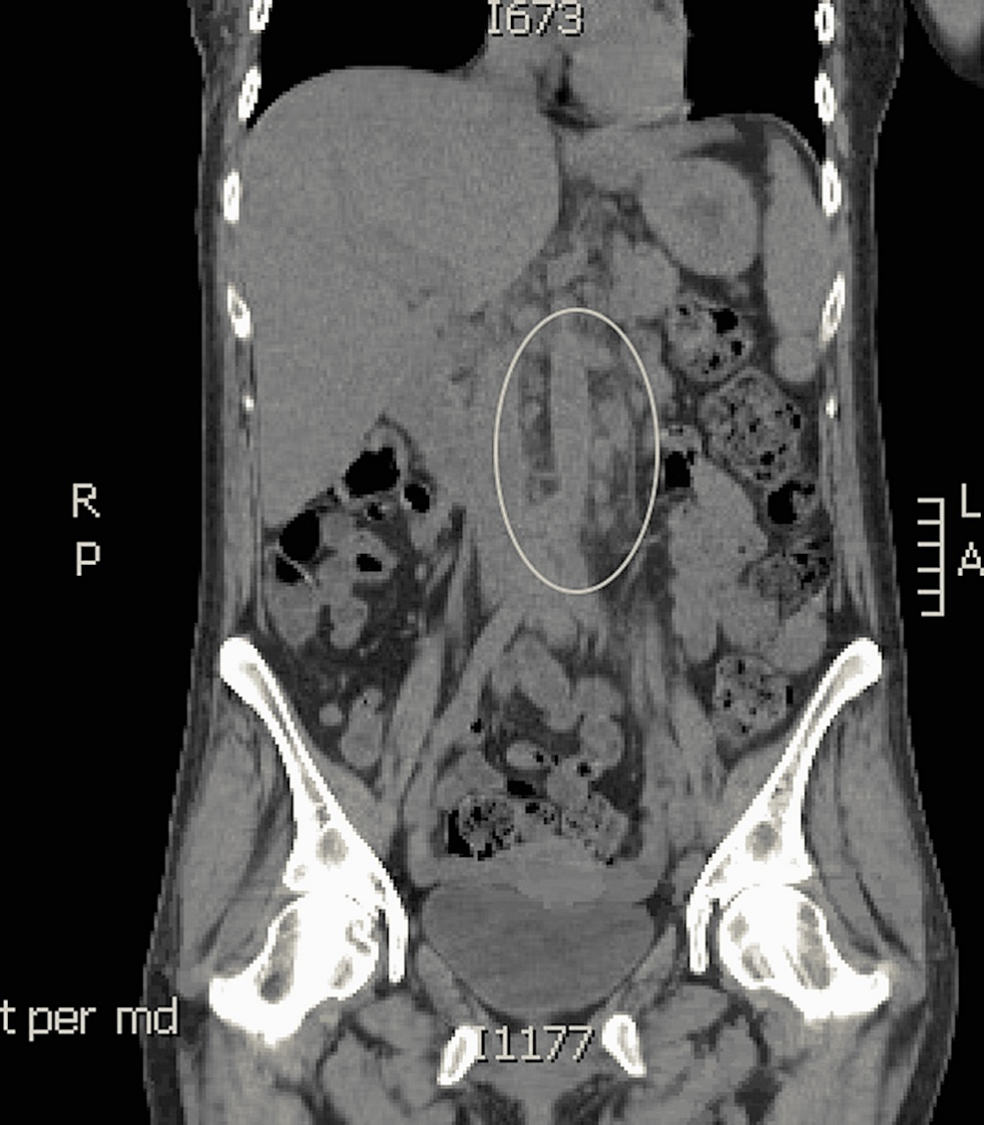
A computed tomography scan of the abdomen and pelvis. The peri-aortic and retroperitoneal lymph nodes are circled.
